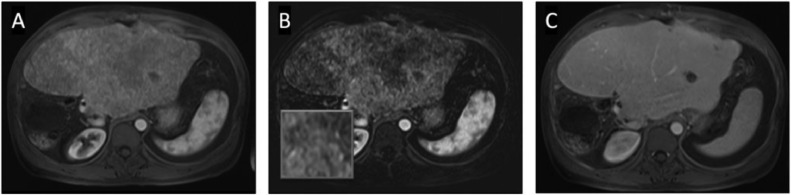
Contrast-enhanced MRI demonstrates heterogeneous arterial parenchymal enhancement (A and subtraction image B) that does not persist on delayed imaging (C).
